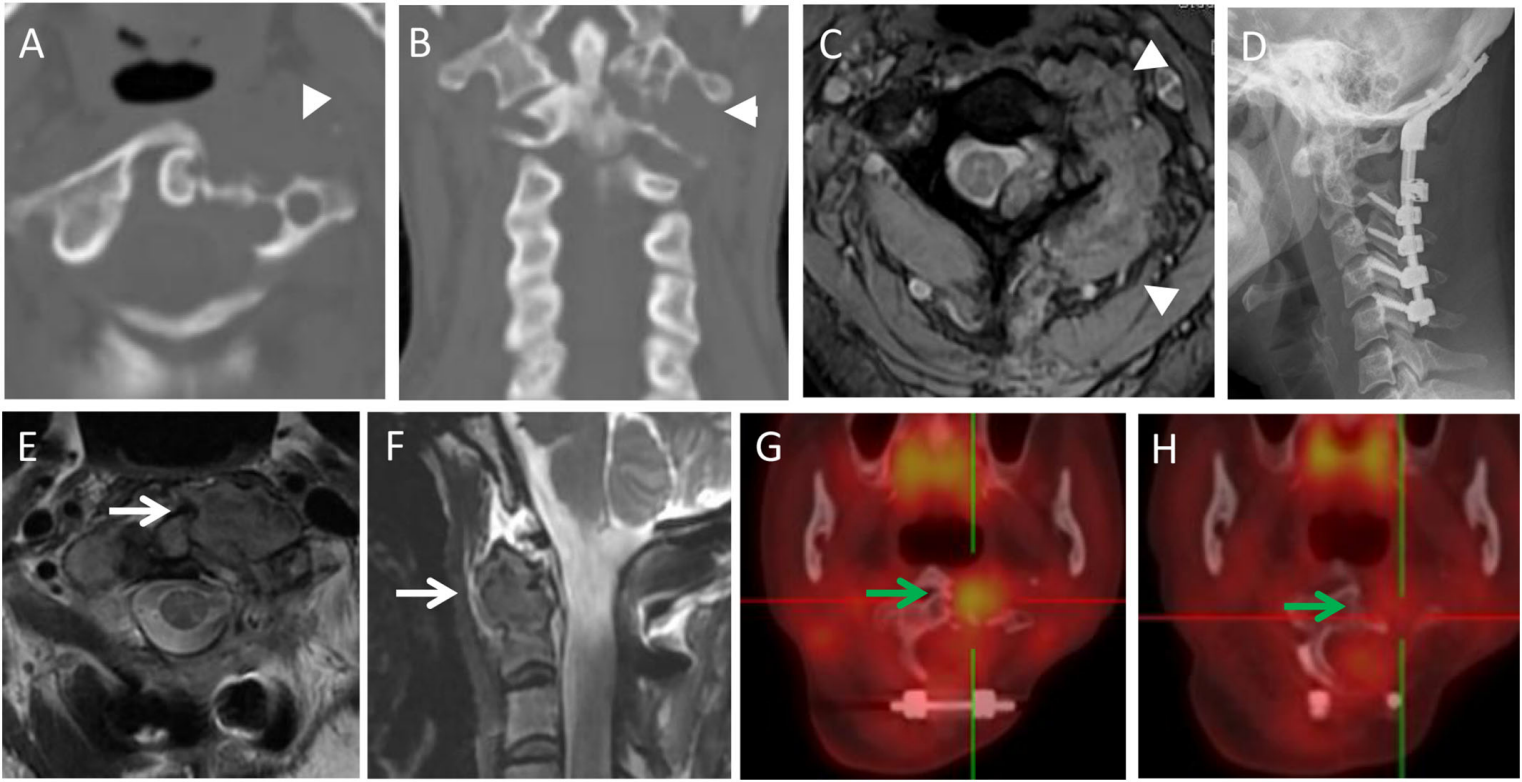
A 44-year-old female with neck pain for five months, an osteolytic lesion was located in the C1-2 (arrowheads). She underwent STR because of vertebral artery invasion. In 6th month follow-up, the MRI scans revealed the local recurrence with a soft tissue mass (white arrows). She underwent radiotherapy but refused further operation. In the subsequent 96 months follow-up, the lesion was stable and repeated PET-CT revealed the reduced uptake of the lesion (green arrows). (A,B): Preoperative axial and coronal CT scans; (C): Multiecho gradient-echo sequence axial MRI scan; (D): Postoperative X-ray; (E,F): T2-weighted axial and sagittal MRI scans in 6th month follow-up; (G): PET-CT in 18-month follow-up after radiotherapy (SUV max 6.0); (H): PET-CT in the 90-month follow-up after radiotherapy (SUV max 2.1).
